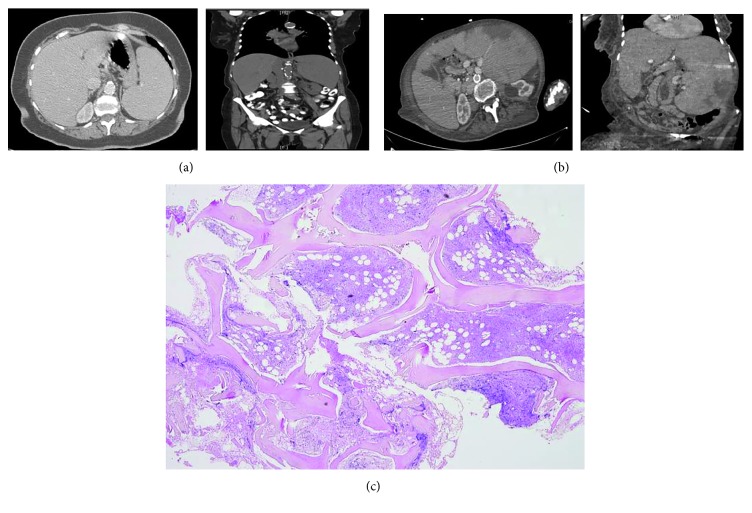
CT scan showing (a) 15 cm splenomegaly after CLL diagnosis in 2006. (b) CT scan in 2013 showing increased massive splenomegaly (28 cm) with splenic infarct and hepatomegaly, after PMF diagnosis. (c) Bone marrow biopsy showing hypercellular bone marrow with fibrosis, consistent with the diagnosis of myelofibrosis.
